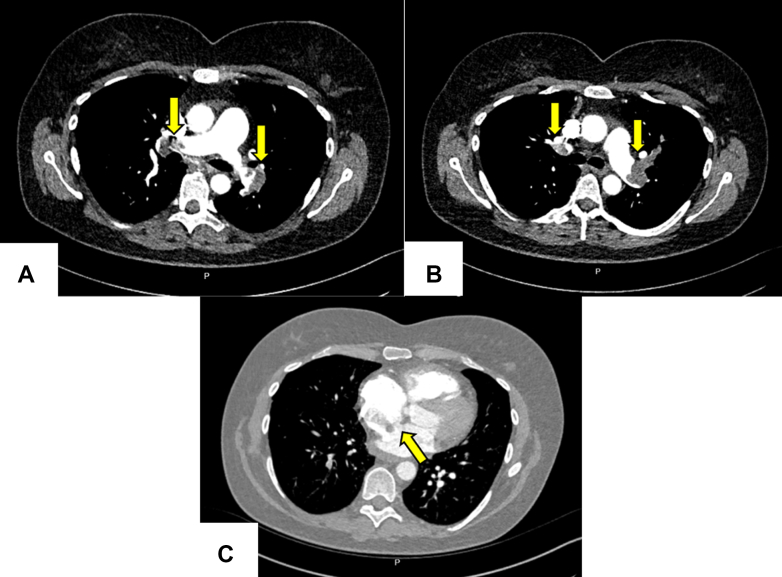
Initial Findings on Computed Tomography (A) The occlusive thrombus in the right and left main pulmonary arteries is shown just before their division (arrows). The main pulmonary artery is clear of thrombus. (B) The occlusive thrombus in the left main pulmonary artery is shown (arrows) extending to totally occlude the left upper lobe branch. (C) The patent foramen ovale is shown, with thrombus visible in the right atrium (arrow).
